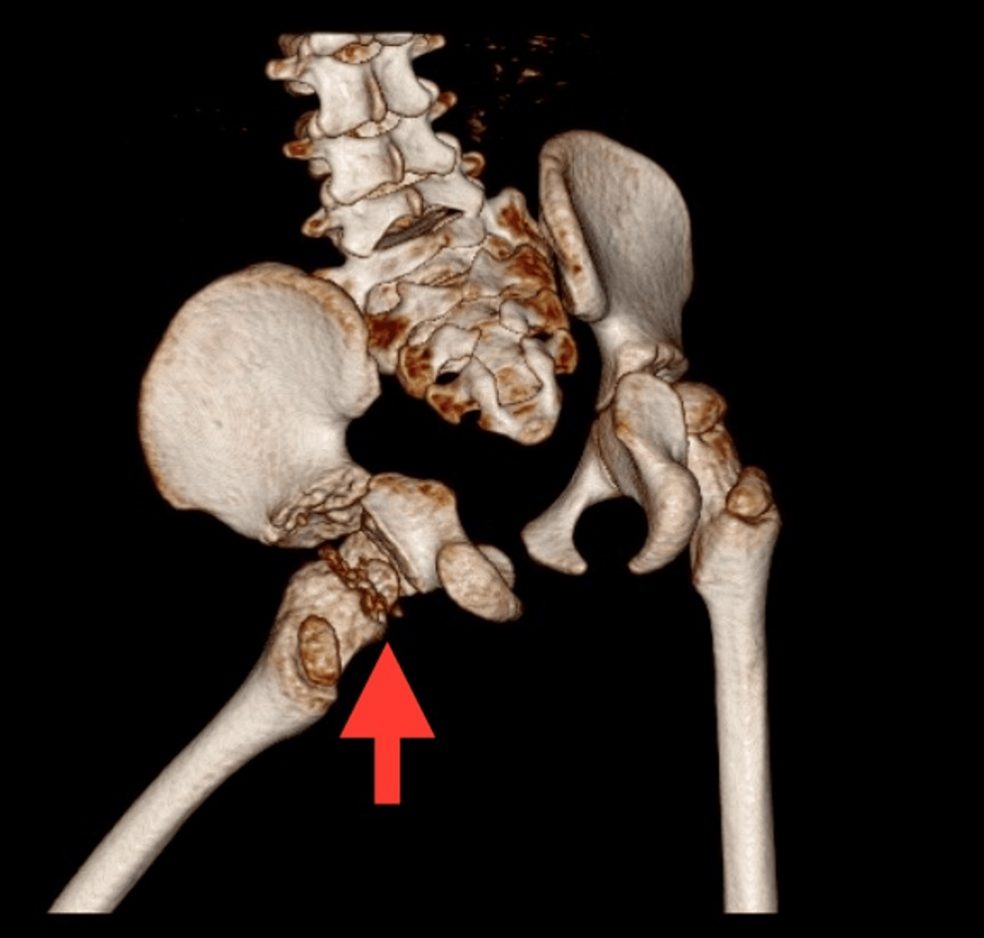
3D CT scan of the posterior view of the left hip joint 3D: three dimensional; CT: computed tomography
